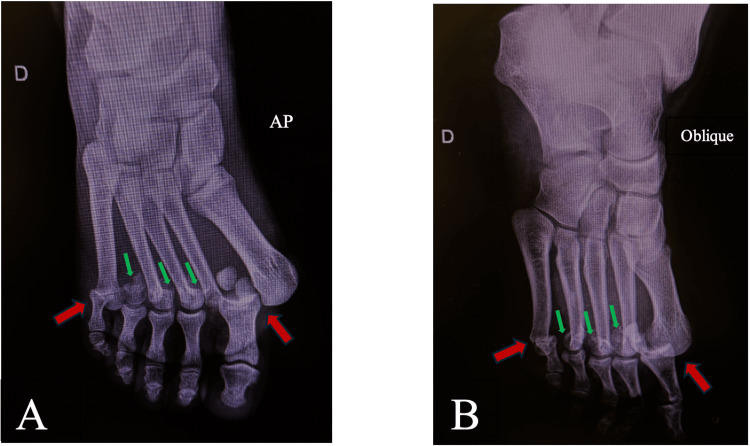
AP (A) and oblique (B) initial right foot radiographs showing lateral dislocations of the first and fifth MTP joints (red arrows) and neck fractures of the second, third, and fourth metatarsals (green arrows) MTP, metatarsophalangeal
